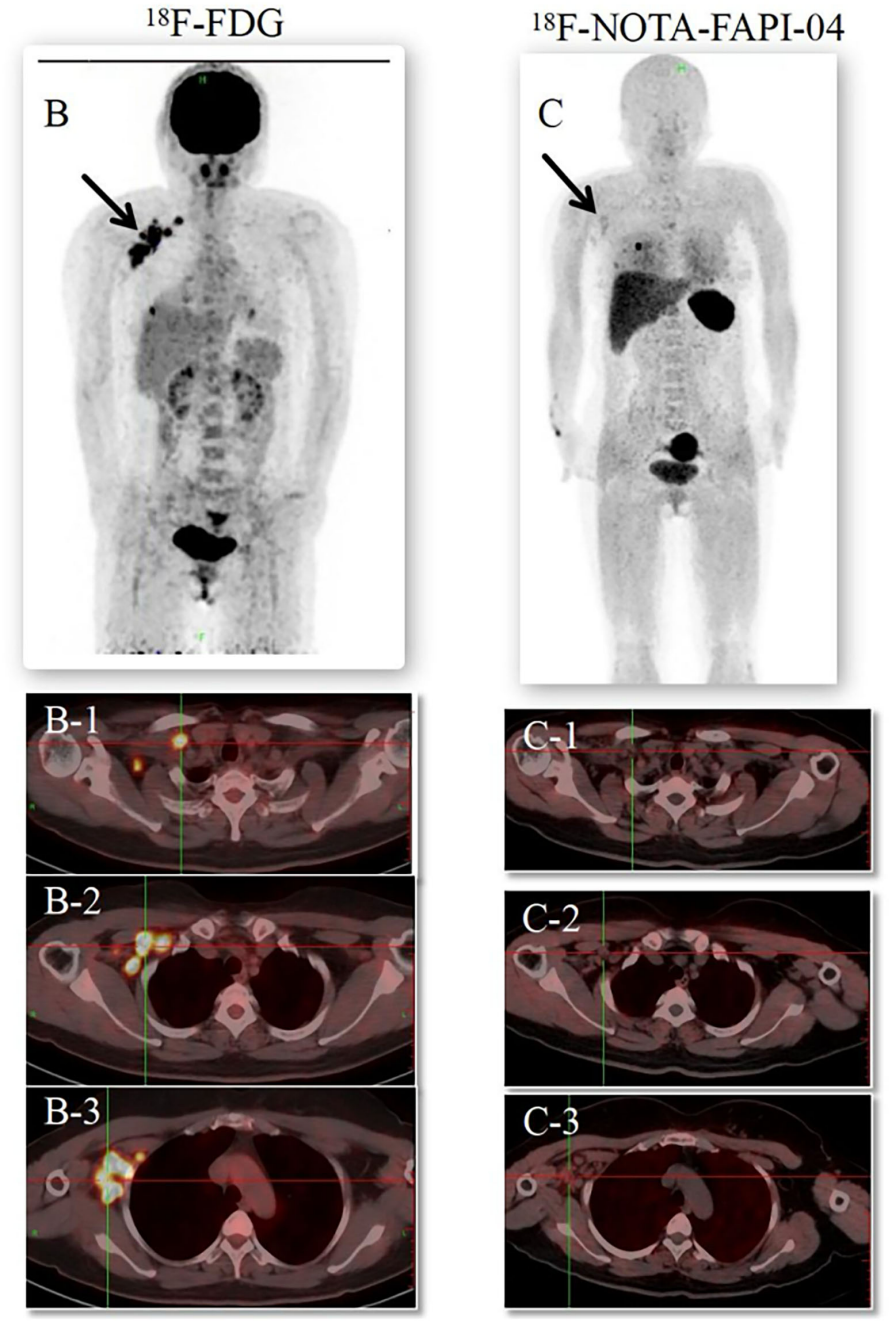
Comparison of [^18^F]‐FDG and [^18^F]‐NOTA‐FAPI‐04 PET/CT.
